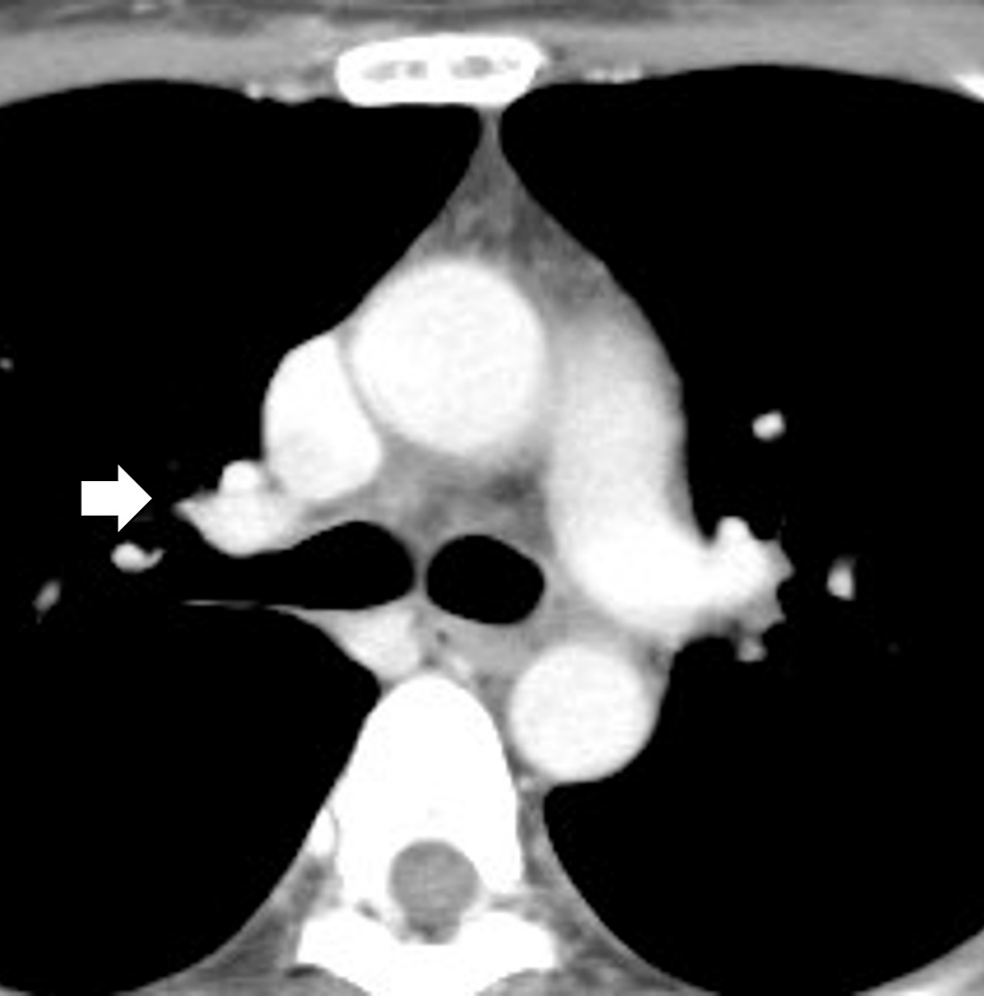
Computed tomography scanning of the chest revealing a remarkable improvement of hilar and longitudinal lymphadenopathy 13 months after the first visit
